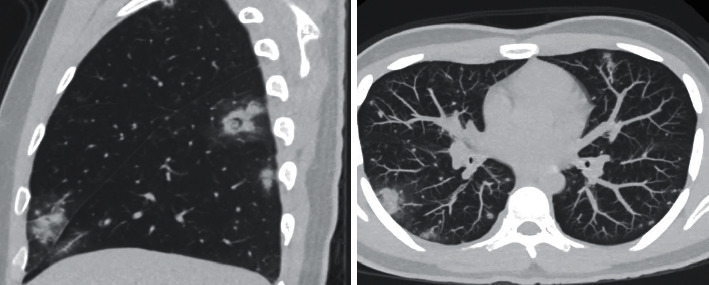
Sagittal and axial MIP CT reconstructions demonstrate numerous round centrilobular nodules, with the larger nodules demonstrating areas of central necrosis and peripheral ground glass opacity.
